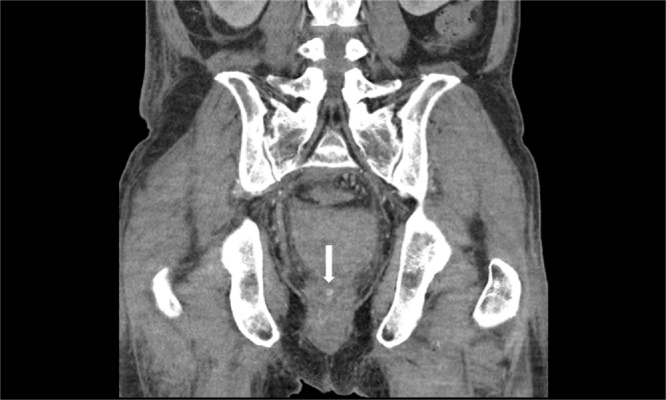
Follow-up contrast-enhanced coronal computed tomography image showing the residual pseudoaneurysm in the rectum (arrow).
